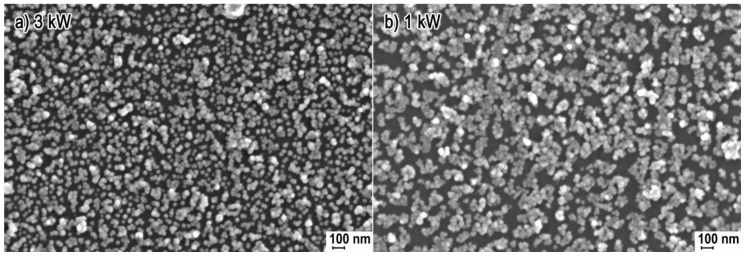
SEM images of ZnO NPs layers obtained on polyurethane film using the ultrasonic method from NPs: (a) ZnO-3 kW; (b) ZnO-1 kW.
